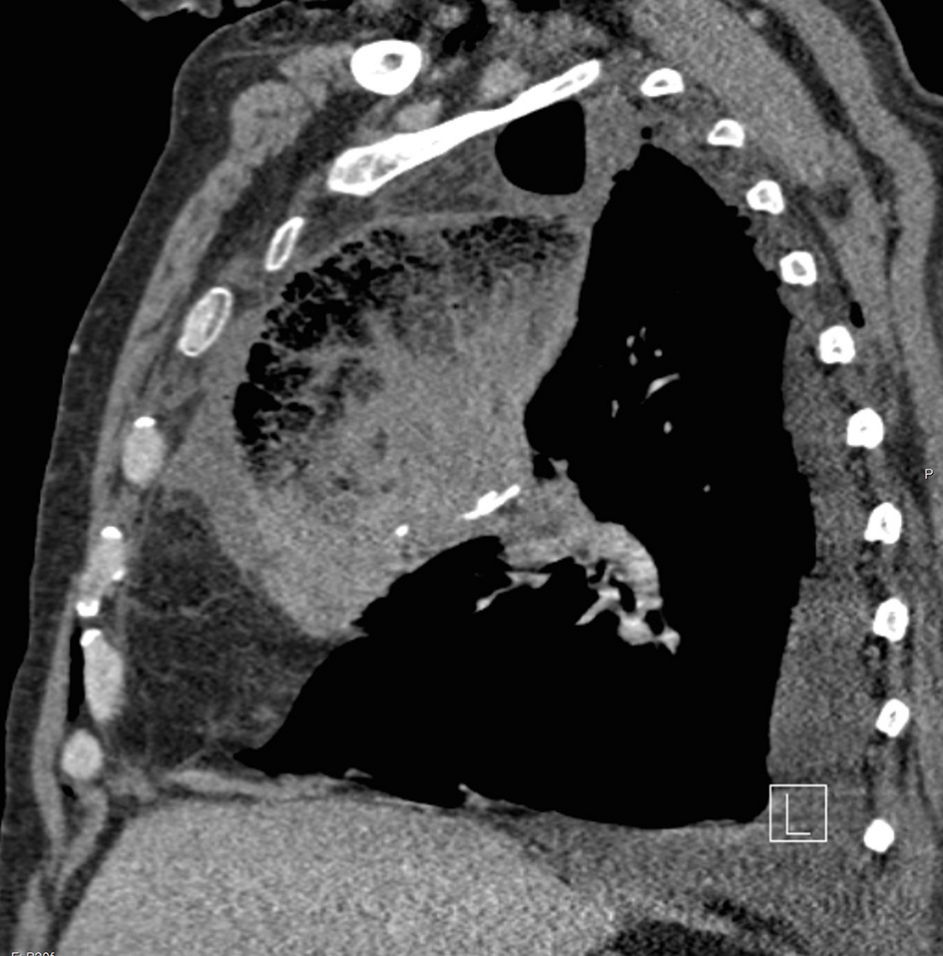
Sagittal reformat of the post-operative contrast-enhanced CT scan on soft tissue window. The middle lobe can be seen tapering towards the hilum and hilar surgical clips. There is interlobular septal thickening and expansion of the poorly enhancing superiorly positioned middle lobe.
